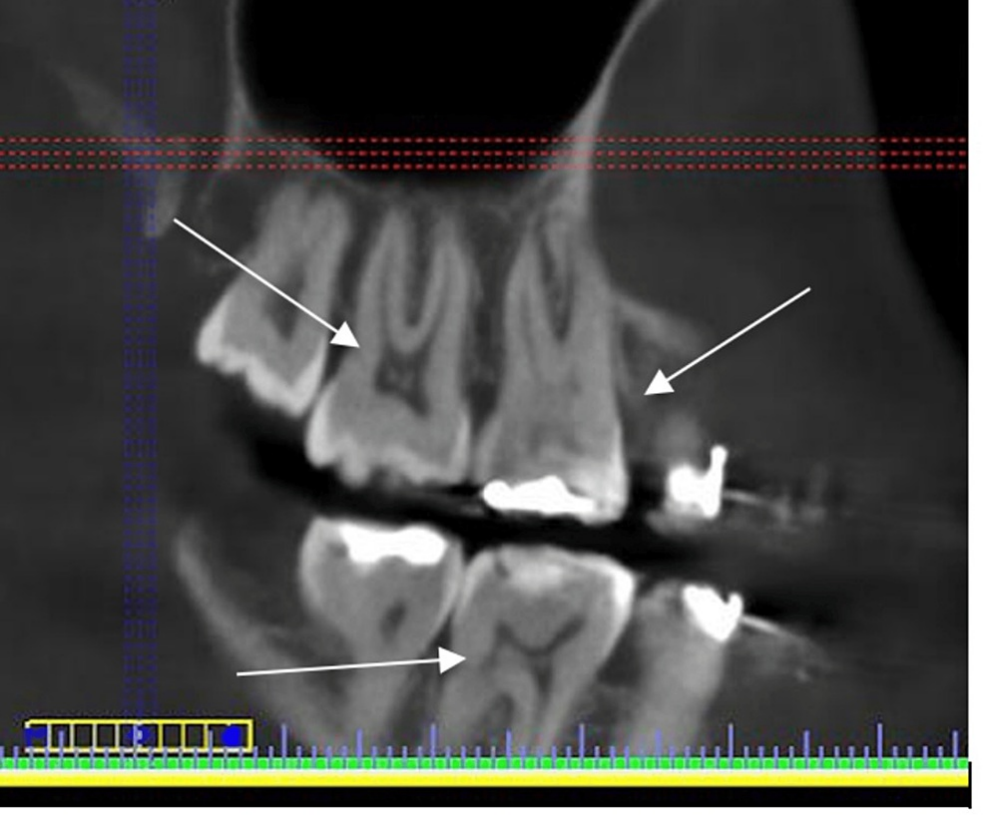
CBCT sagittal section showing pulp stones in the upper first and second molars and lower first molar CBCT: cone-beam computed tomography
